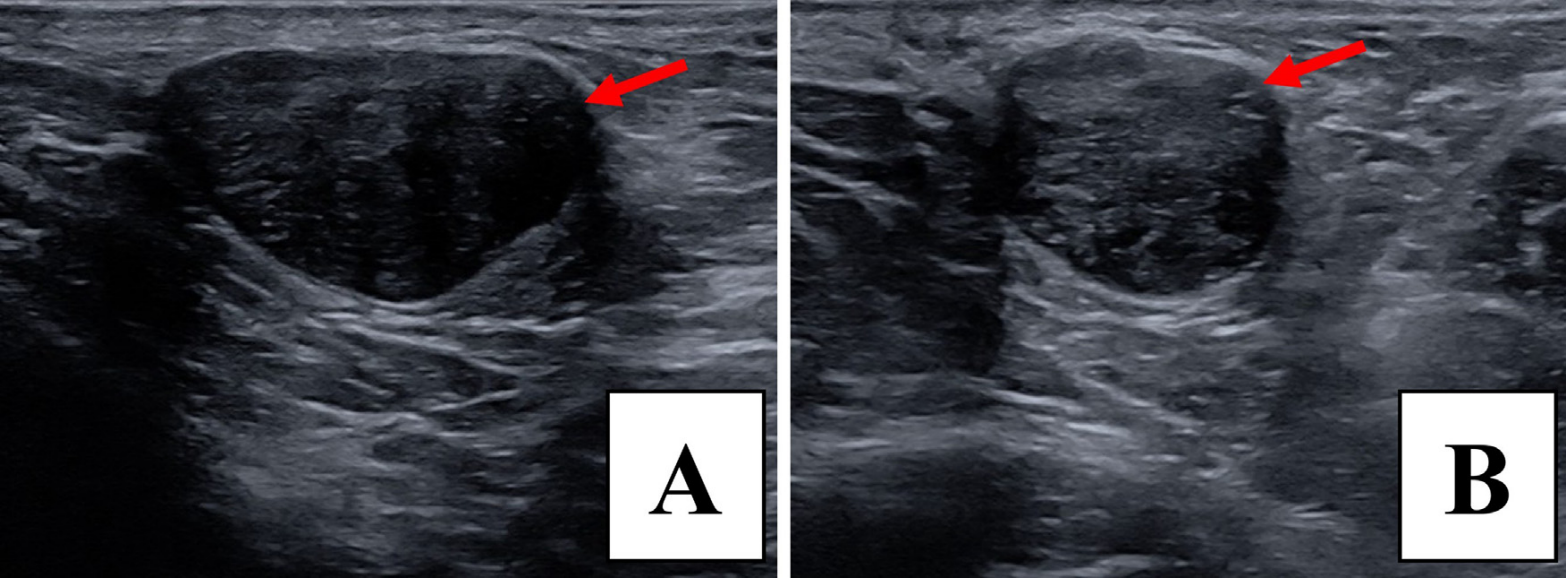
Ultrasound imaging demonstrates an oval, circumscribed, hypoechoic 2.8 × 1.6 × 1.9 cm mass in the right axilla in sagittal (A) and transverse (B) views, with red arrows highlighting the lesion.
